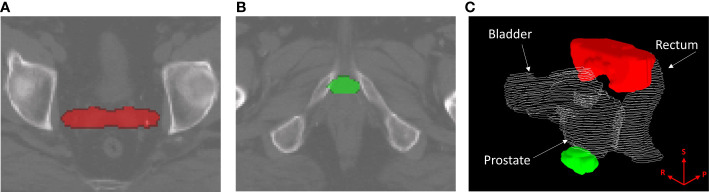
Regions where the dose distribution was significantly different (p ≤ 0.02) between patients who did and did-not experience biochemical recurrence (BCR) within four years of radiotherapy, for patients treated with intensity modulated radiotherapy plus a single high dose rate brachytherapy boost. Regions are overlayed on the planning CT of the reference patient used for image registration and dose mapping, and indicate where excess dose was associated with lower BCR. The identified region in (A), marked in red, corresponds with the seminal vesicle tips. The region in (B), marked in green, corresponds to the apex region of the prostate. (C) shows the two regions in relation to the prostate, rectum and bladder of the reference patient. The superior (S), right (R) and posterior (P) directions are indicated on the render in (C).
